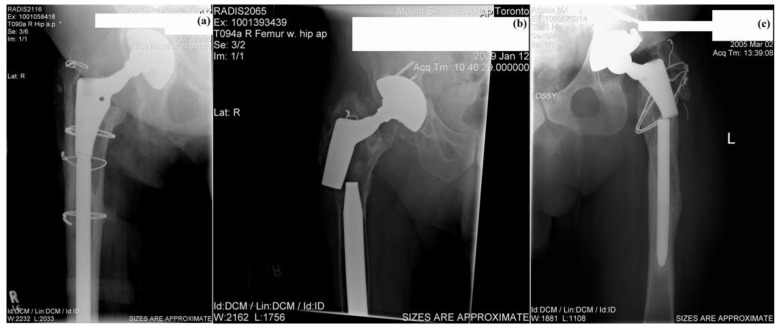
Radiographs revealing the fractures in vivo of cementless femoral stems in three humans (a–c) represent cases 1, 2 and 6, respectively, in Table I in Ref. [266]). The failures were initiated by a fretting fatigue mechanism and propagated via pure bending fatigue [266]. Reprinted with permission from Wolters Kluwer Health, Inc.
